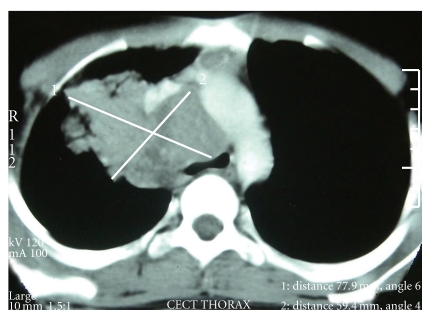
CT scan of the chest is showing a homogenous mass measuring 7.5 cm × 6.0 cm. The mass is compressing the trachea causing significant lumen compromise.
